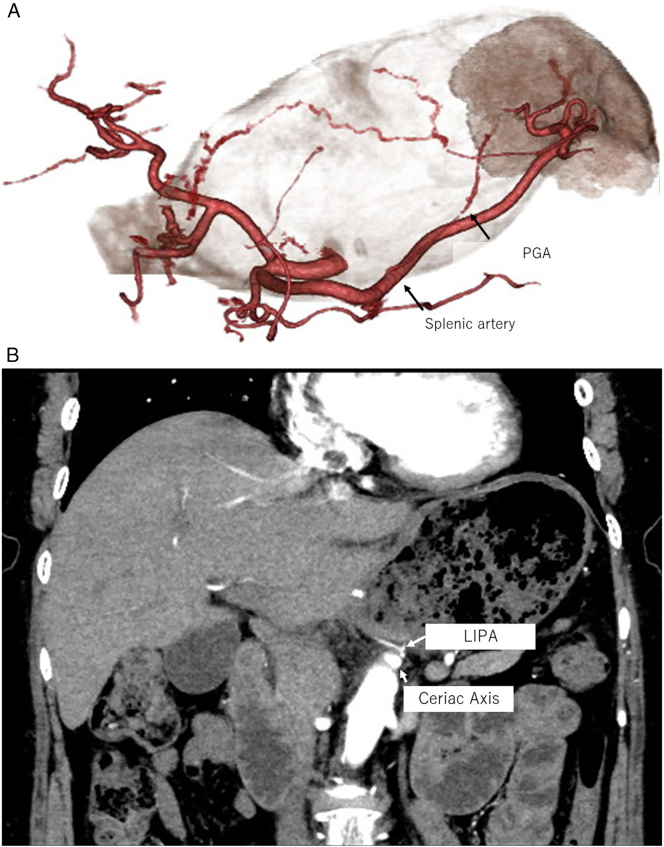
CT angiography; (A) three-dimensional CT imaging before splenectomy showed a developed PGA. (B) Arterial phase CT showed the LIPA branched from the celiac axis. LIPA, left inferior phrenic artery.
